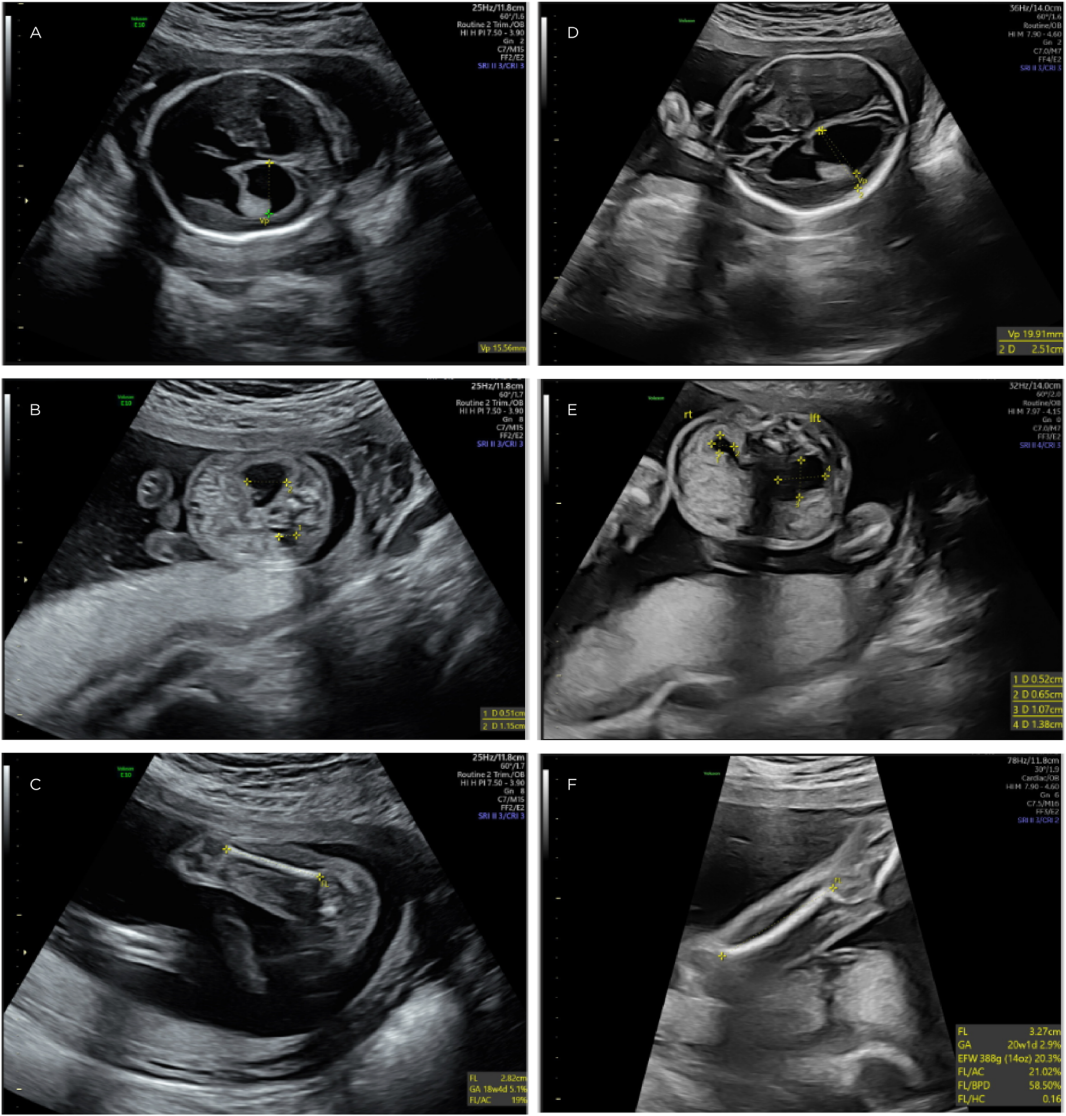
20-week anatomy ultrasound findings compared to 22-week follow-up ultrasound. Transabdominal ultrasound images showing severe bilateral ventriculomegaly (A), renal pyelectasis (B), and short femur (C) length at time of 20-week anatomy ultrasound (left) and worsening in severity at a follow-up 22-week ultrasound (D–F, respectively) (right).
